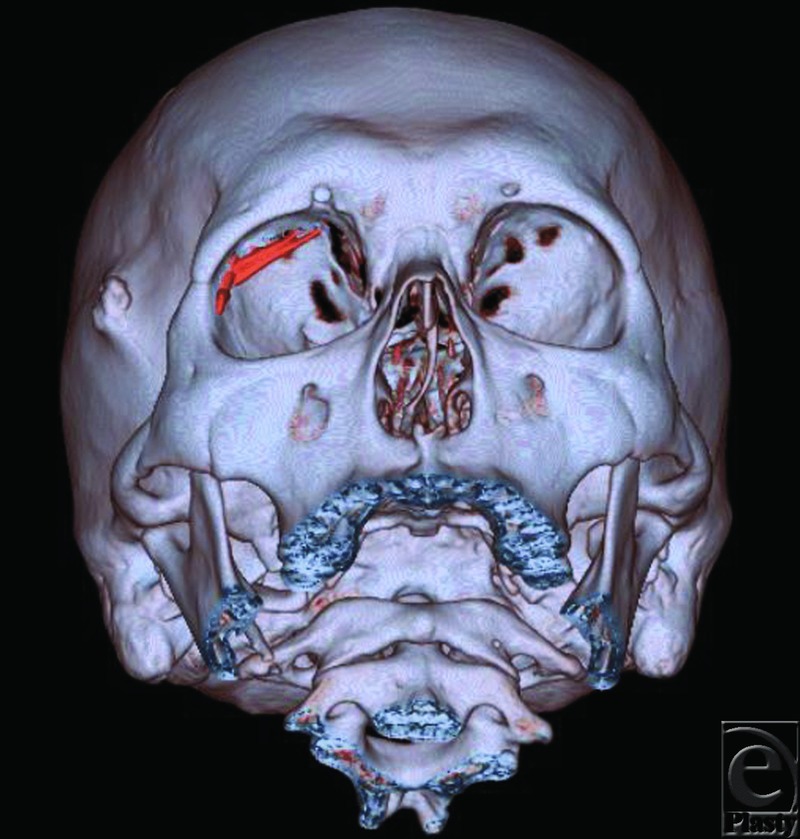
CT indicating calcifications in the upper part and outer side of the right orbit. The elongated objects depicted in red are the foreign bodies.
